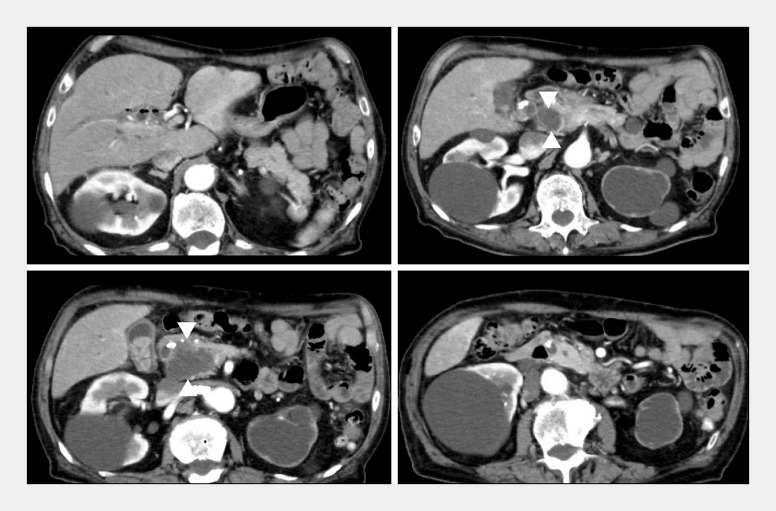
Computed tomography showed that bile duct dilation was improved by a biliary stent. However, the pancreatic cyst had not decreased in size (arrowheads).
